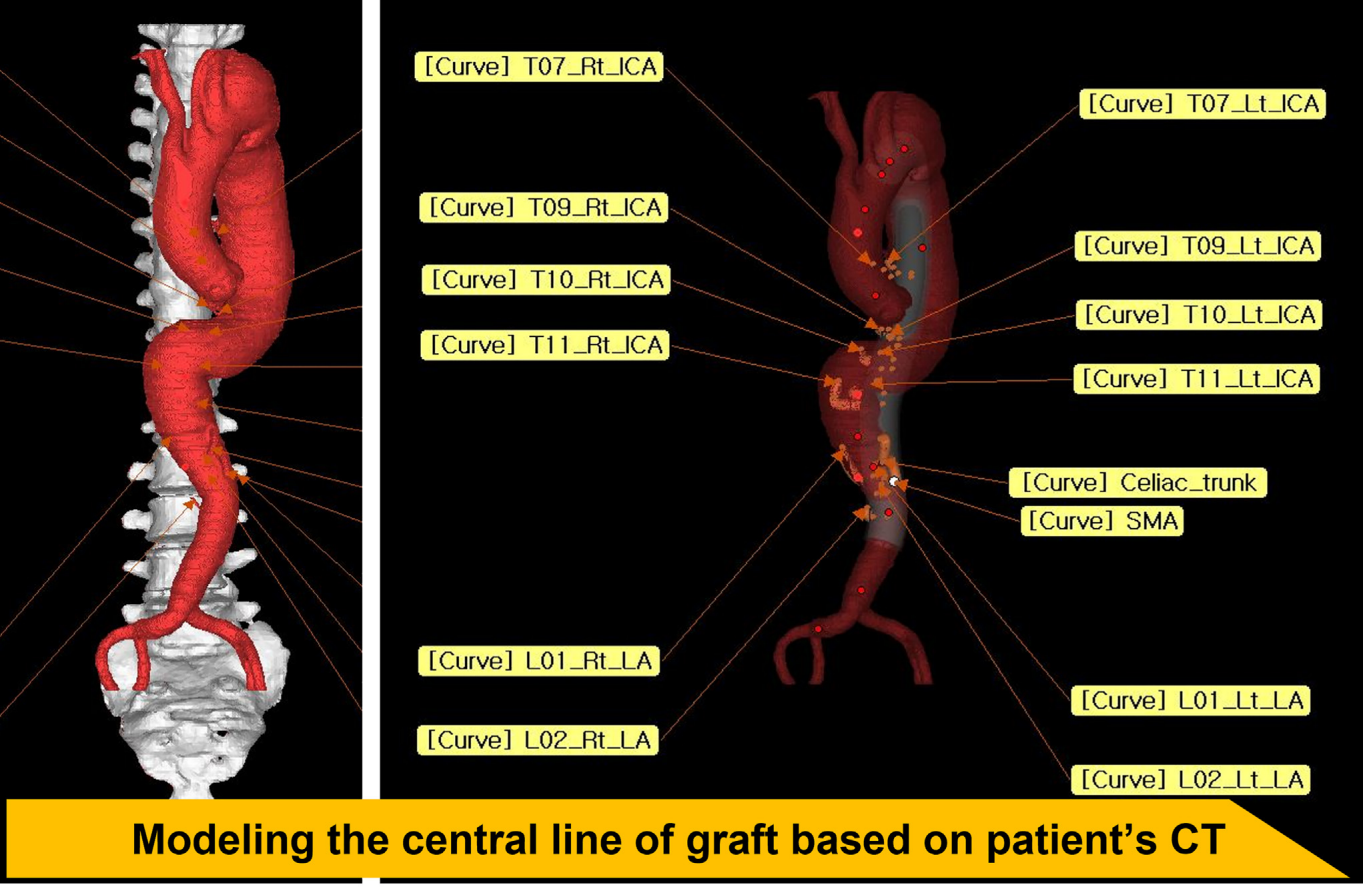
Three-dimensional modeling process for the aortic graft framework. *Rt*, Right; *ICA*, intecostal artery; *Lt*, left; *SMA*, superior mesenteric artery; *LA*, lumbar artery; *CT*, computed tomography.2.The final version of the 3D aortic graft model incorporating segmental and visceral arteries was mutually created by the operator and 3DP manufacturer (Anymedi Inc) (Figure 1).3.The aortic graft model was printed out as 2 guides (visualization and marking) (Figure 2, *A*). It takes approximately 3 days to 2 weeks from acquiring the patient's CT image to receiving the 3D-printed models, and the required period can be adjusted according to the patient's emergency. The marking guide was inserted into the TAAA graft while manually constructing the octopod graft, so that the spatial relationship between the branch vessels can be easily and comfortably identified using a small protuberance (Figure 2, *B*). The details of the printing technology are described in our previous publications.^8^^,^^9^
